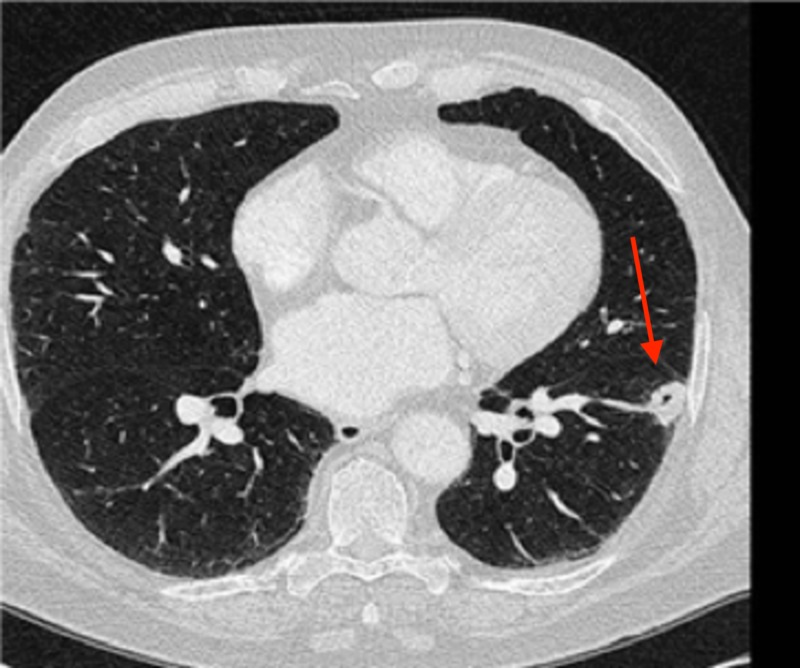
The regression of the untreated left lower lobe lung tumors is shown, with axial slice from the baseline computed tomography (CT) chest shown in Figure 1; an axial slide from three months later revealed a 2.2 x 1.6 cm tumor
